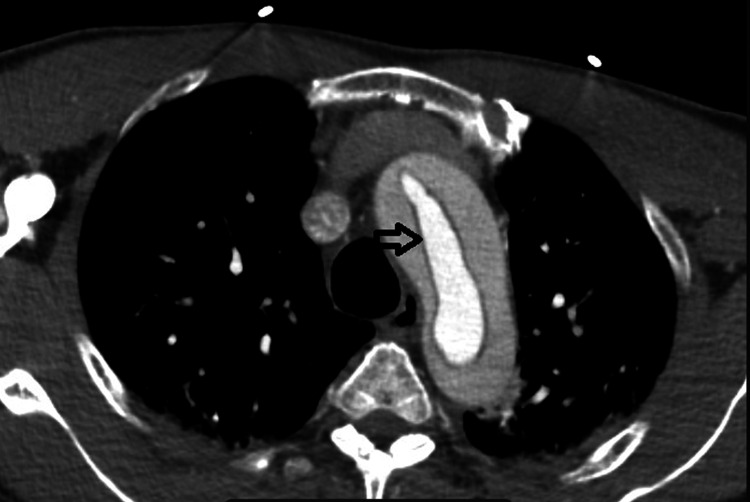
Axial CT angiography image at the level of the aortic arch showing a circumferential oval flap (windsock sign) involving the aortic arch (black arrow) results from intimo-intimal intussusception between the true and false dissected lumens.
